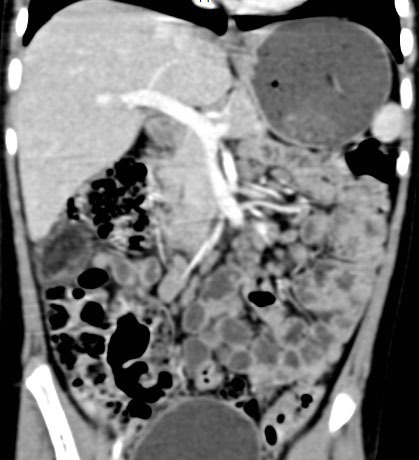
Figure 1: Presence of fatty component along with enhancing soft tissue is seen within the well-defined, encapsulated mass lesion on CT scan.
